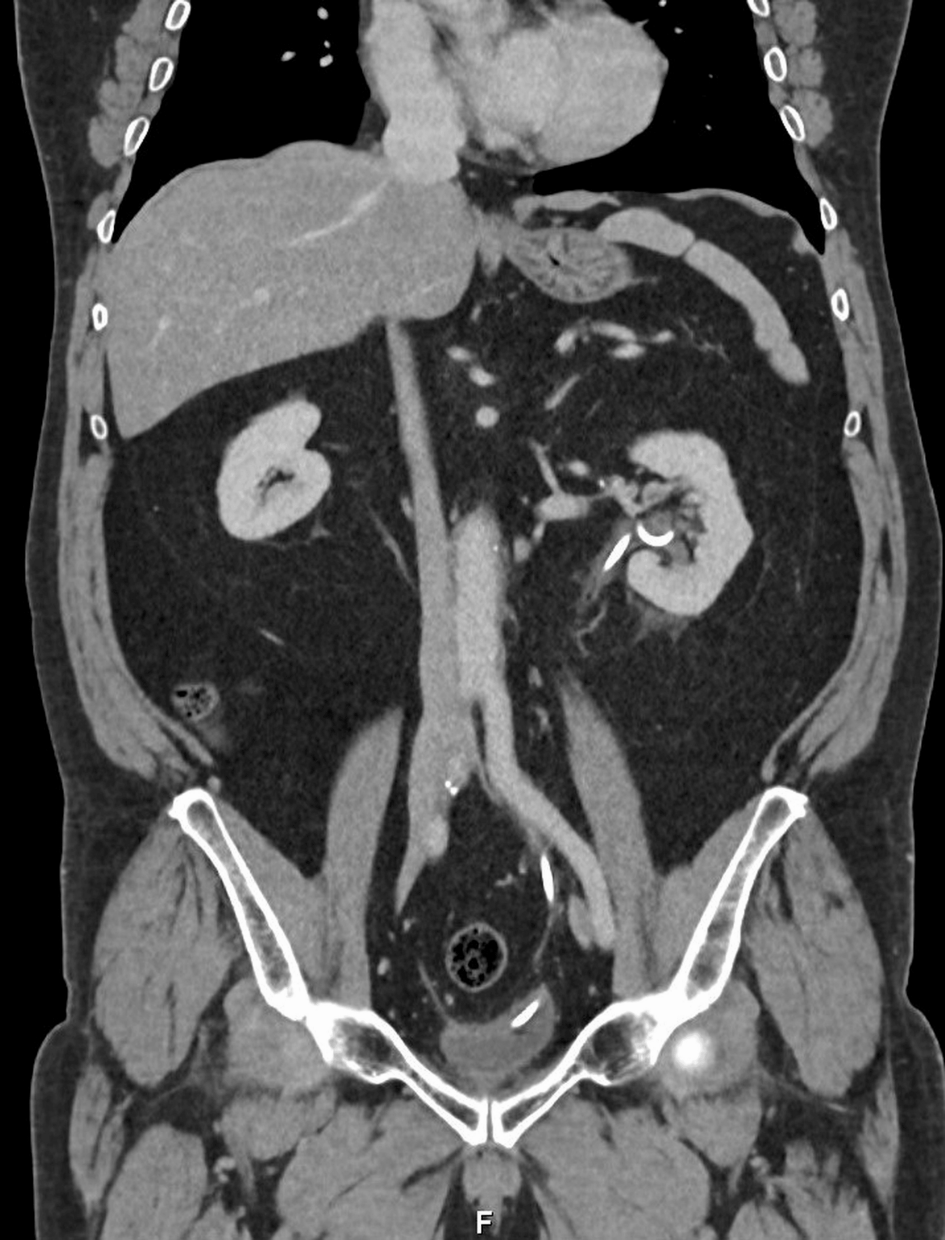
Follow-up abdominal and pelvic contrast-enhanced CT Follow-up contrast-enhanced abdominal and pelvic computed tomography (coronal view) demonstrating complete resolution of the previously described retroperitoneal soft-tissue thickening, with no residual ureteral obstruction.
